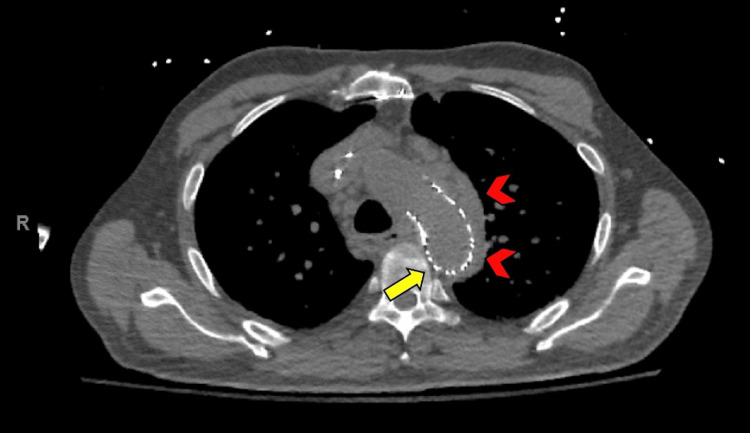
Axial CT cut demonstrating the covering of the lesion with the stent graft (yellow arrow), preventing hematoma enlargement (red arrowheads).
